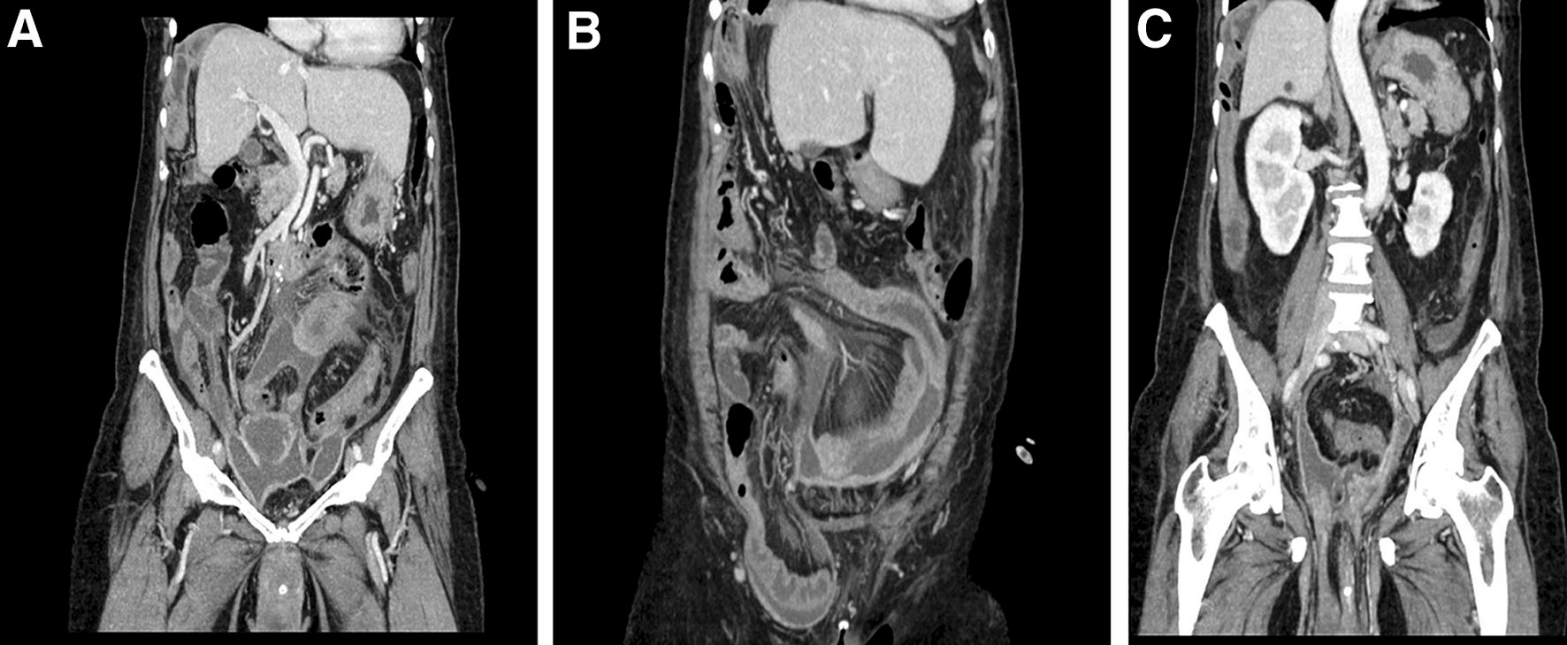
Computed tomography (CT) findings at admission. Coronial view shows massive ascites and a cystic mass in the lower-right abdomen that ruptured to abdominal cavity (a). CT also revealed a right inguinal hernia containing the small intestine (b) and uterine prolapse (c)
